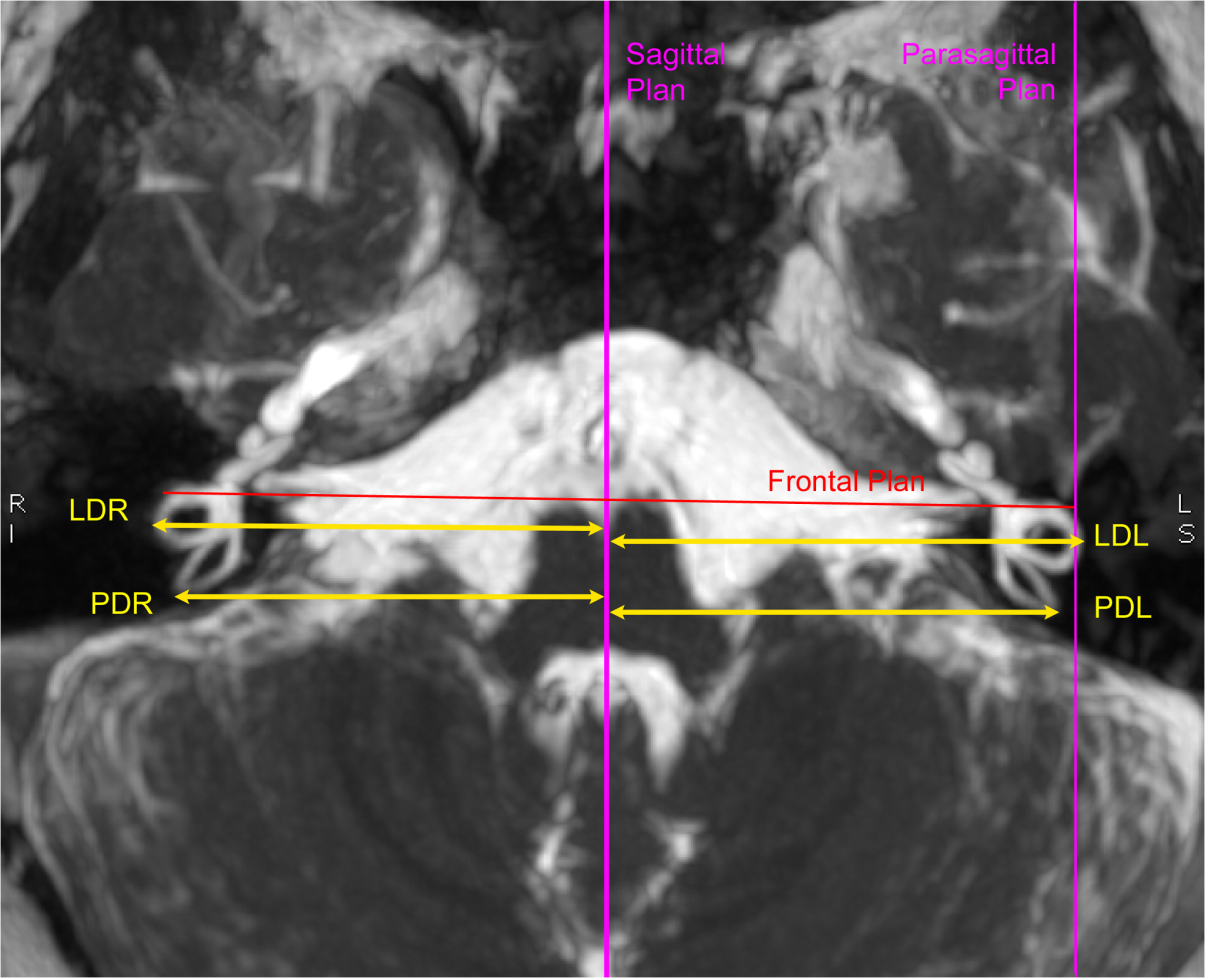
Definition of planes and semicircular canal position measurements. T2 3D MRI showing sagittal, parasagittal, and frontal planes. Distances of the semicircular canal are defined as: “*lateral distance right*” (LDR) for the right lateral canal, “*lateral distance left*” (LDL) for the left lateral canal, “*posterior distance right*” for the right posterior canal (PDR), and “*posterior distance left*” (PDL) for the left posterior canal.
